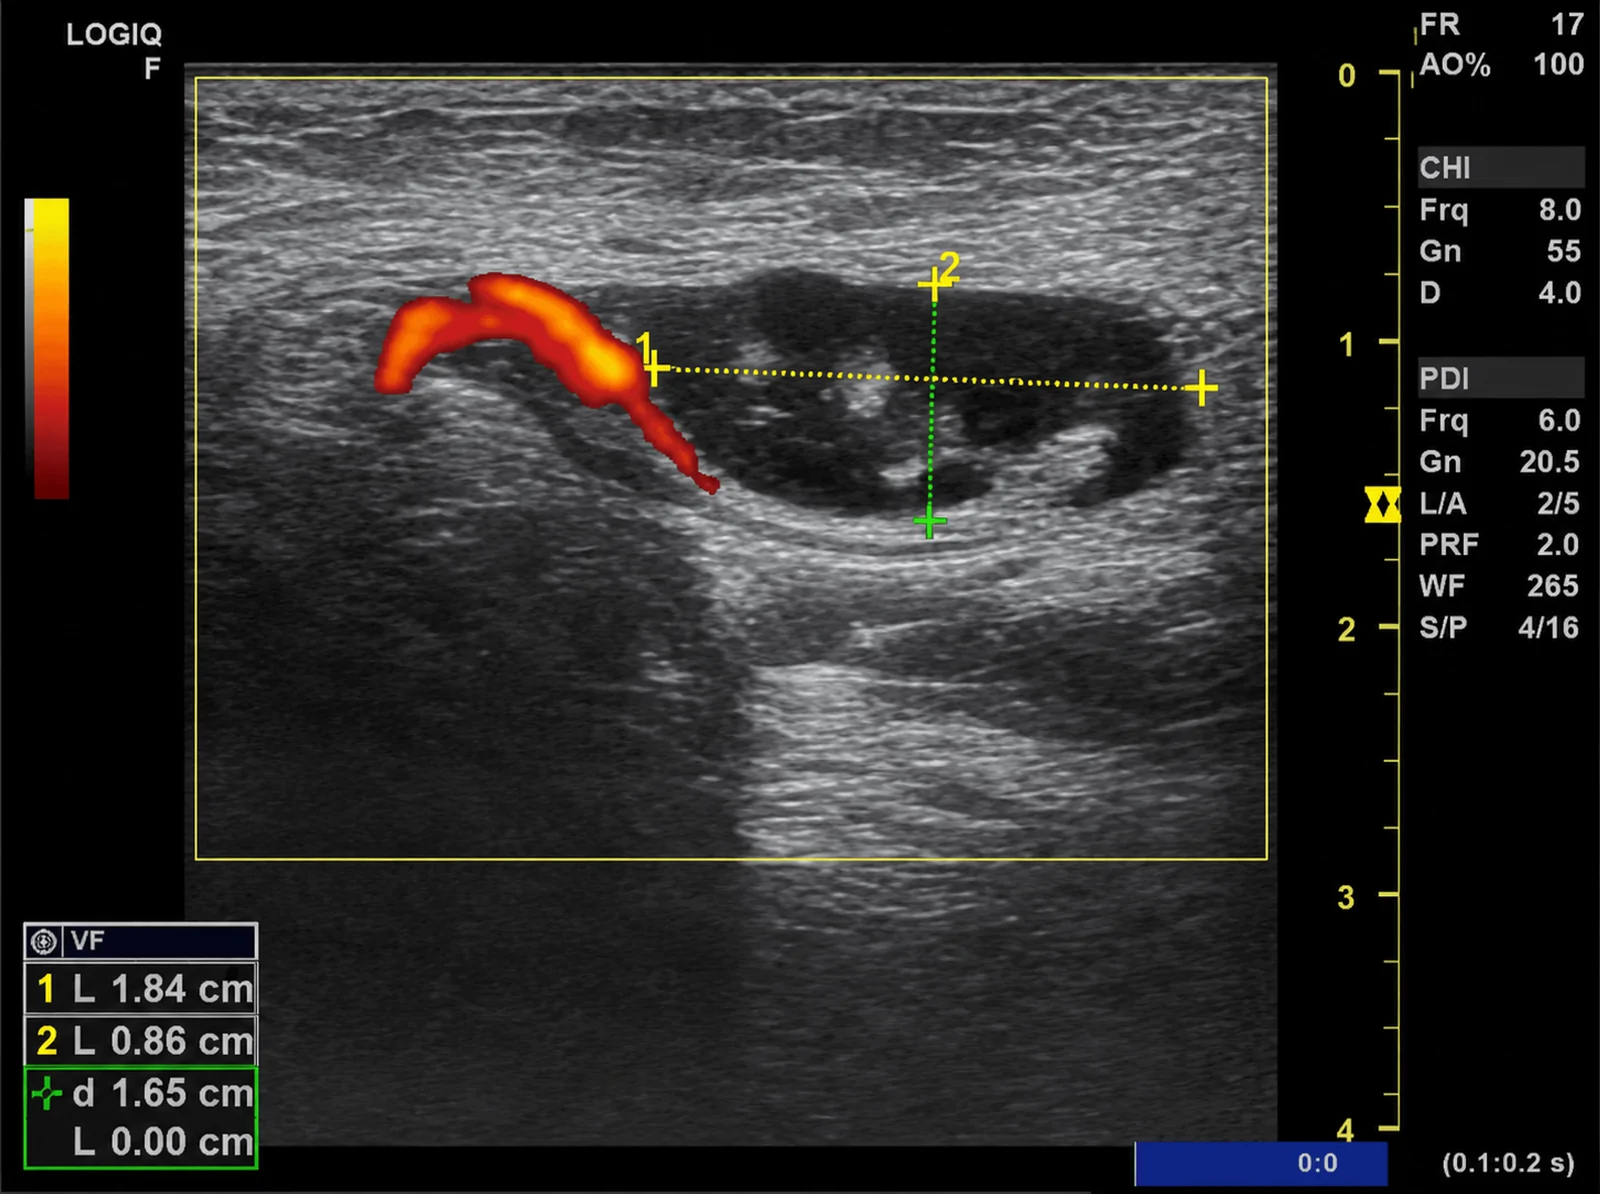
Case one - color Doppler ultrasonography revealed a well-defined hypoechoic area measuring about 1.84 cm x 1.65 cm in the right buccal space
